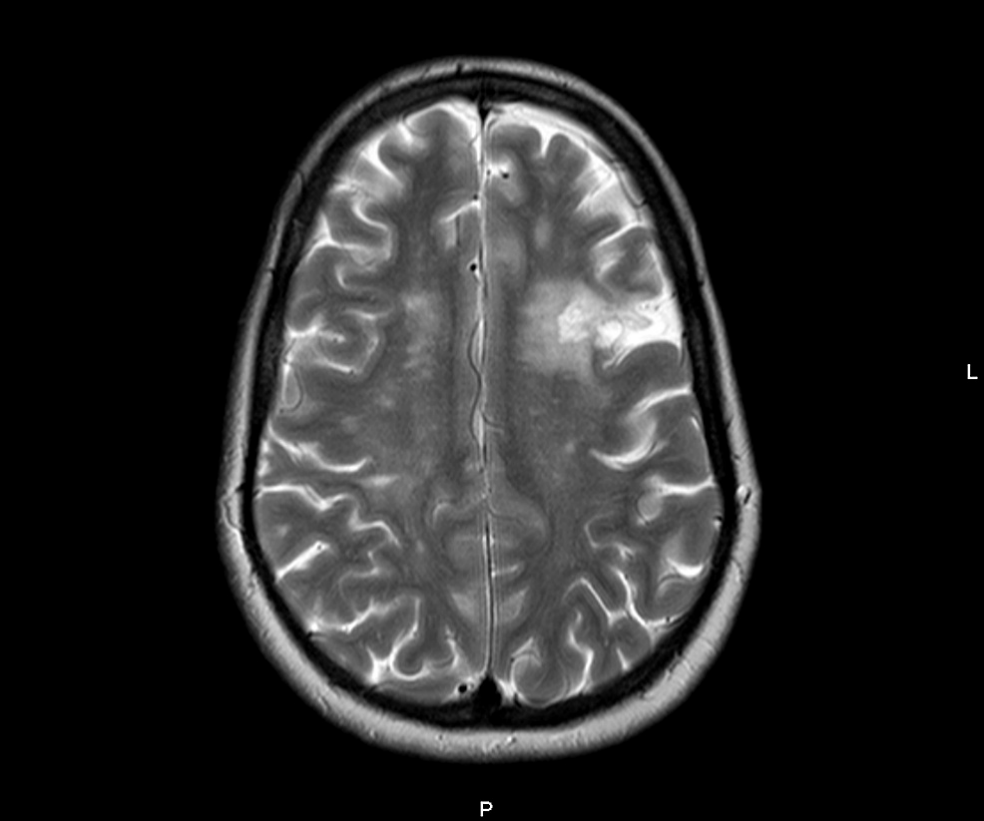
Cerebral magnetic resonance T2-weighted image showing a small recent frontoparietal ischemic lesion
